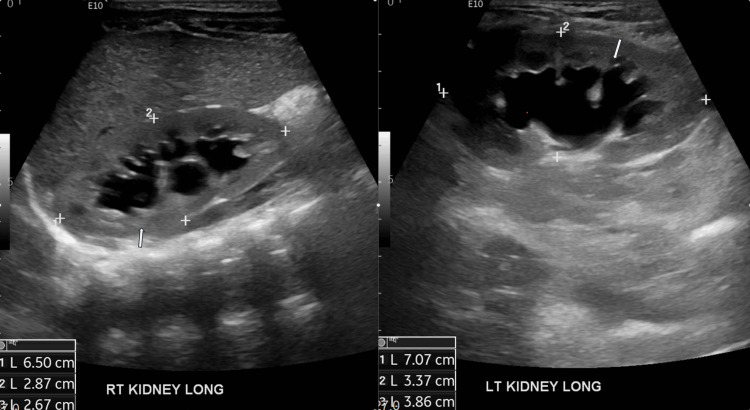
Renal ultrasound showing bilateral mild to moderate hydronephrosis.
